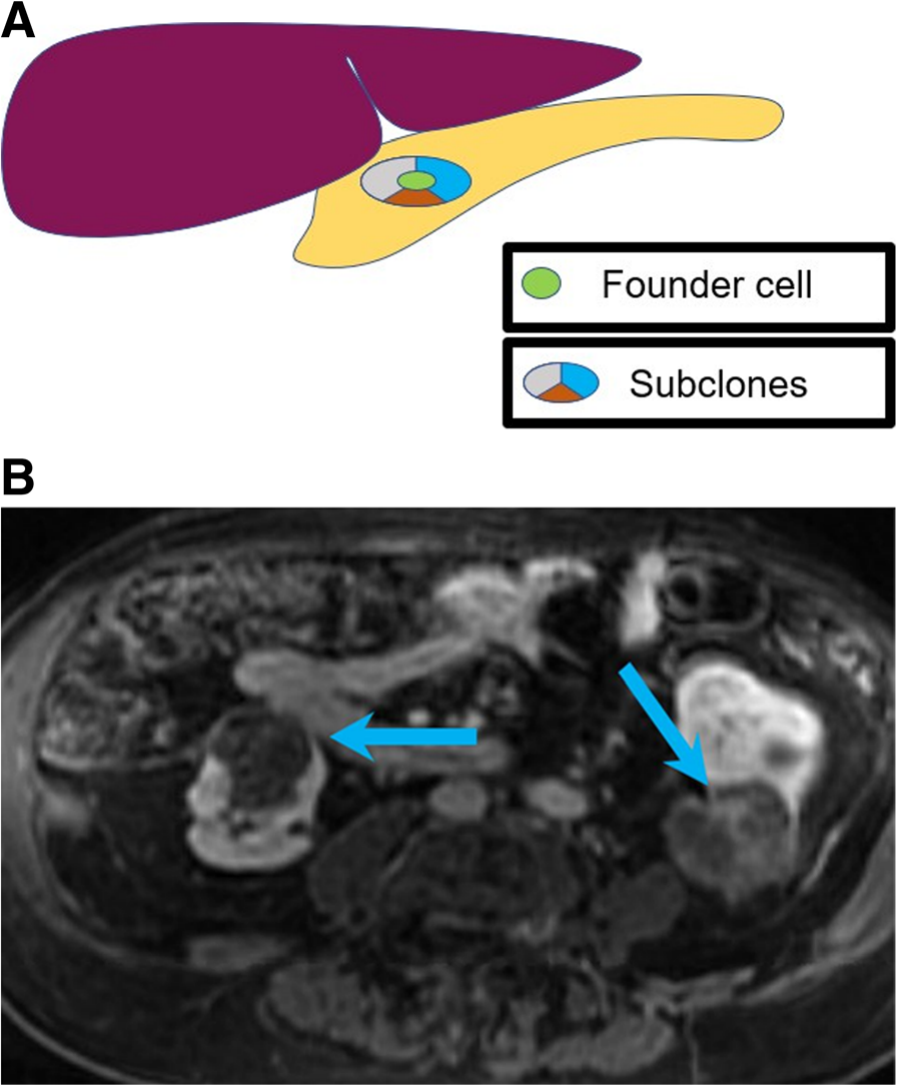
Intratumoral heterogeneity. a Image exemplifying the heterogeneity within the subclonal cells of a pancreatic tumor, all derived from a founder cell. b Fat-saturated T1-weighted postcontrast MRI image acquired during arterial phase showing heterogeneously appearing renal cell carcinomas (blue arrows). Mutations can be missed at tissue sampling obtained from biopsies, given the mutational heterogeneity of renal cell carcinoma
